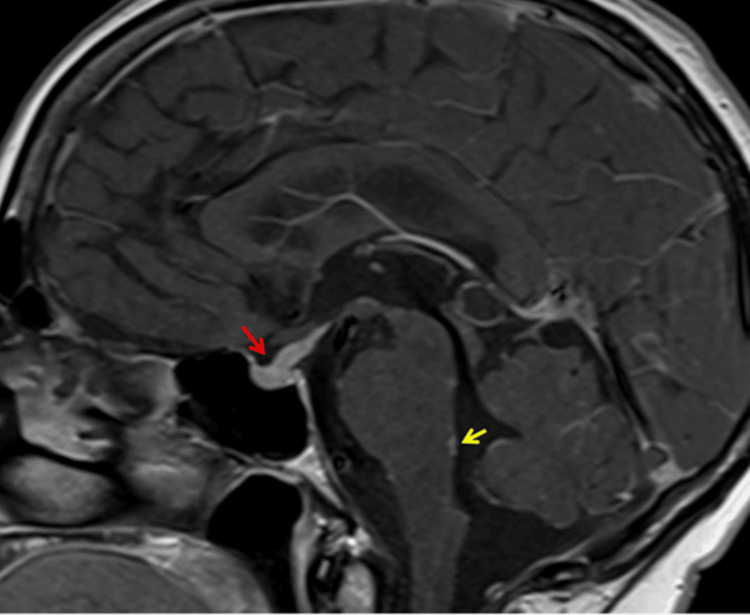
Magnetic resonance imaging of the pituitary Red arrow indicates thickening of the pituitary stalk, and the yellow arrow indicates leptomeningeal post-contrast enhancement.
